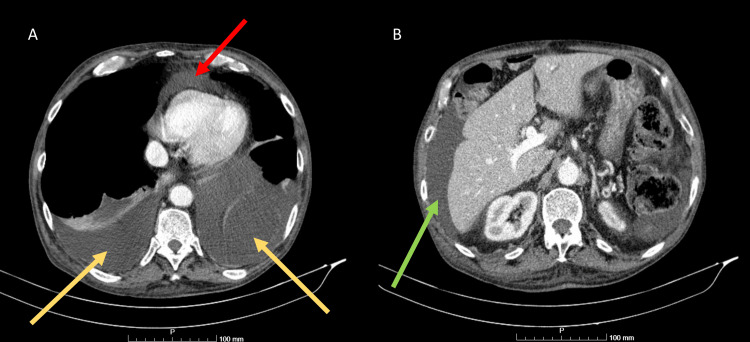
CT-scan A: Thoracic CT-scan: pericardial effusion (red arrow) and pleural effusion (yellow arrows); B: Abdominal CT-scan: ascites (green arrow)
